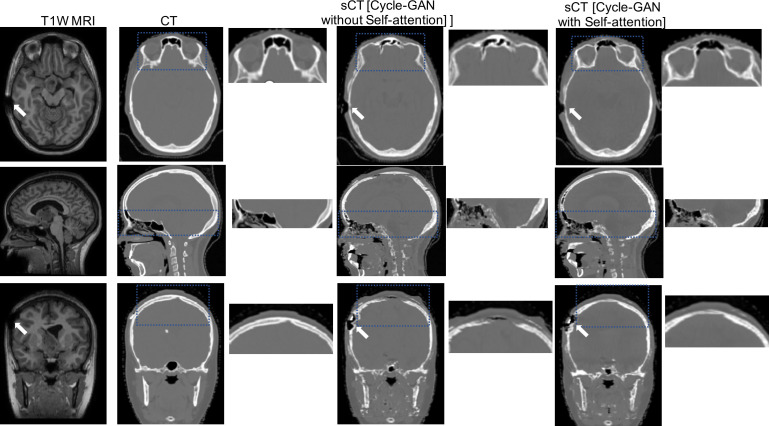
From left to right: T1W MRI_tx_, replanning CT images and its zoom in view, sCT from cycle-GAN without self-attention and its zoom in view, and sCT from cycle-GAN with self-attention and its zoom in view. Abbreviations: CT, computed tomography; cycle-GAN, cycle-generative adversarial network; MRI, magnetic resonance imaging; MRI_tx_, acquired routinely during proton therapy; sCT, synthetic computed tomography; T1W, T1-weighted.
